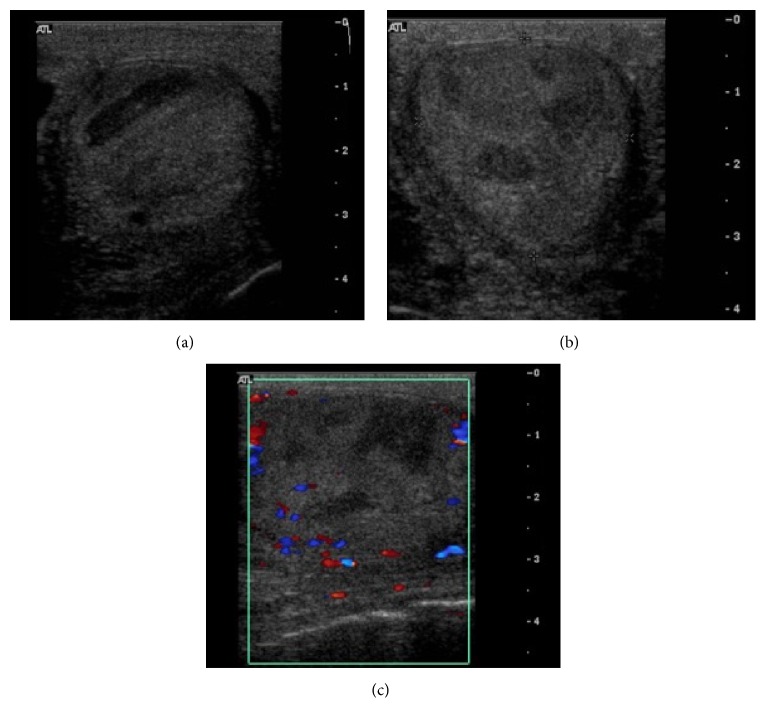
Multiple axial and longitudinal sonographic images (a–c) of the left testicle with Doppler color demonstrating a heterogeneous appearance, with multiple hypoechoic mass-like areas, and lack of Doppler flow within the majority of the testis. The right testis (image not provided) had a similar appearance.
